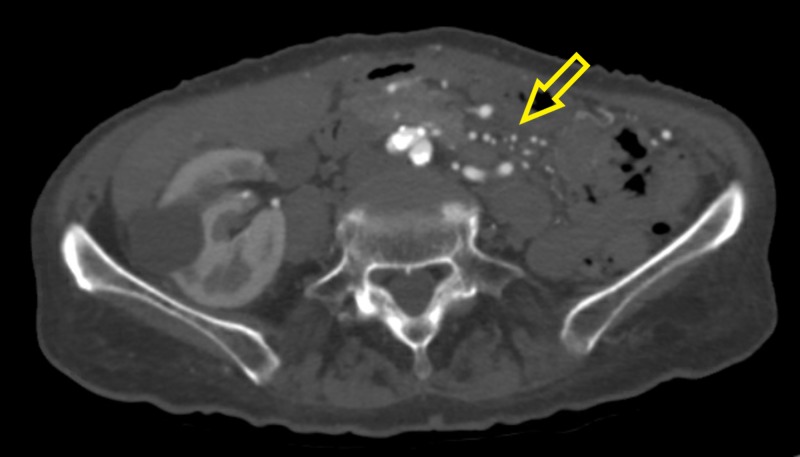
Computed tomography angiography (CTA) of abdomen/pelvis shows inferior mesenteric artery collaterals (yellow arrow)
